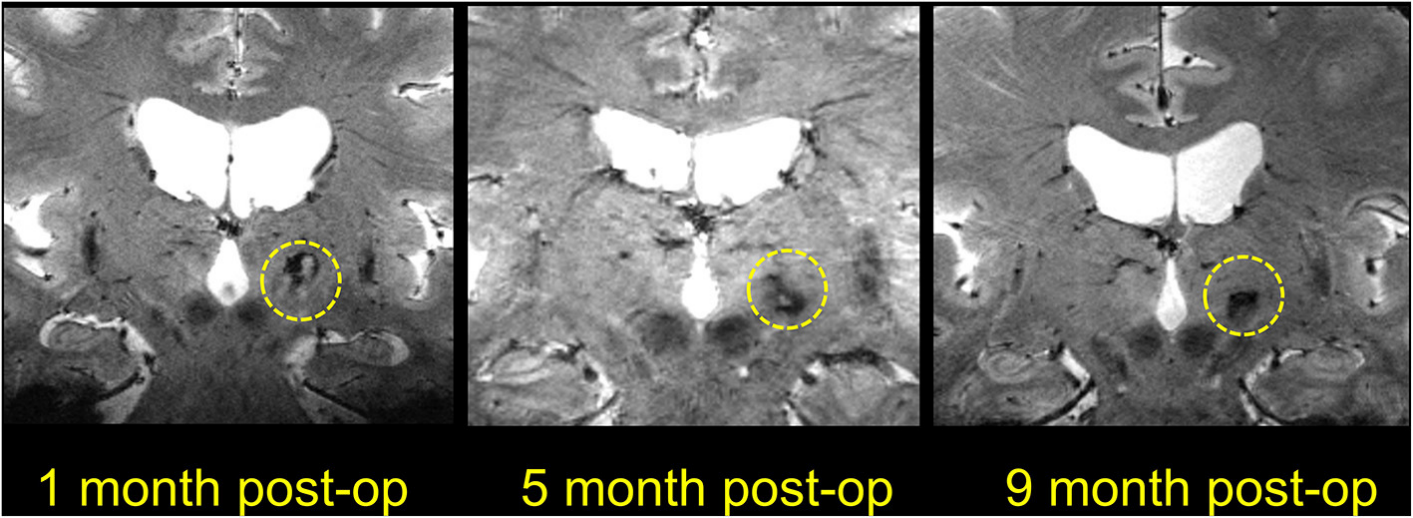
Coronal T1-weighted 7T images showing lesions at different time points after MRgFUS thalamotomy. The three images are all from different patients, as the postoperative MR images were obtained at different time points.
